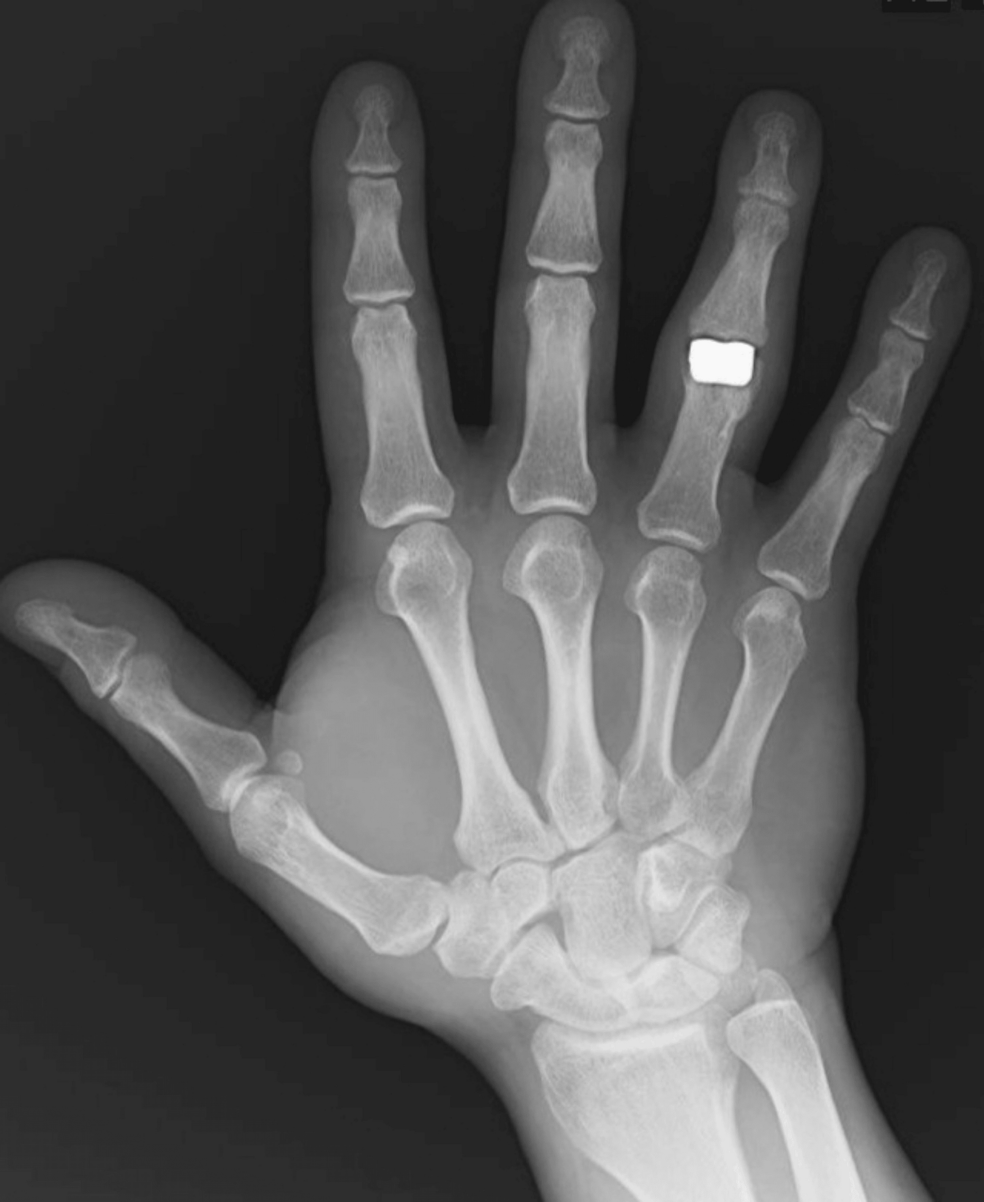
Anteroposterior X-ray obtained eight months after surgery
